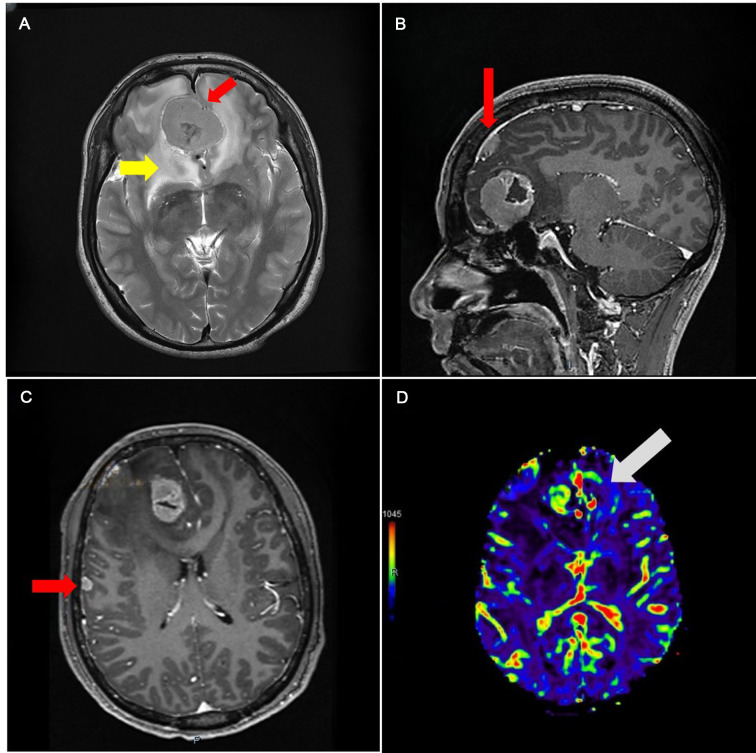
(A) Magnetic resonance image (MRI) image showing isointensity occupancy in T2 series with a clear border compared to the brain tissue (red arrowhead), surrounded by edema (yellow arrowhead). (B) Enhanced series showing the falx (red arrowhead). (C) Lesion with fair enhancement under the right temple bone. (D) CBV showing that the skull base area has more but not fair blood volume.
